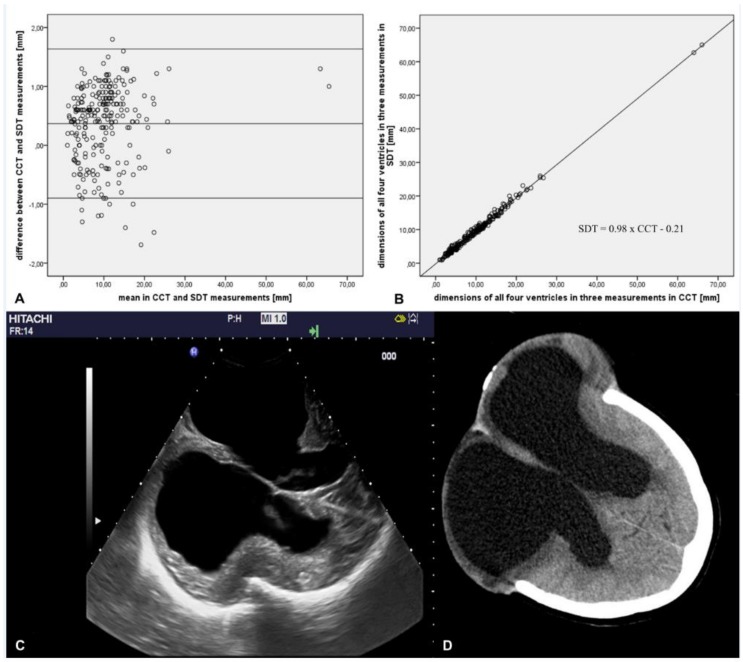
(A–D) Comparison of sonographic duplex technique (SDT) and cranial computed tomography (CCT). (A) Bland Altman test of ventricular size in SDT and CCT (n = 240). (B) Correlation of dimensions of all four ventricles in three measurements in SDT (y-axis) and CCT (x-axis). Linear correlation between the ventricular dimension in SDT and CCT is shown. The formula of the linear regression is SDT = 0.98 x CCT−0.21. Exemplar axial slices for both techniques ((C) SDT and (D) CCT).
